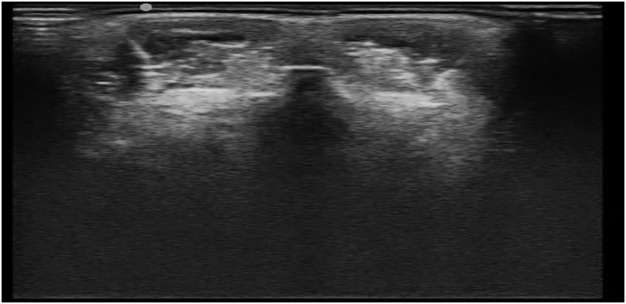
Typical spinal ultrasound image.
